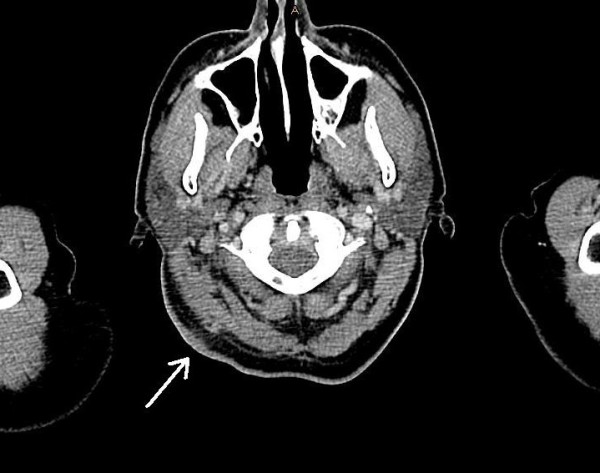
Head computed tomography scan. Imbibition of subcutaneous tissue underneath the eschar (arrow).
